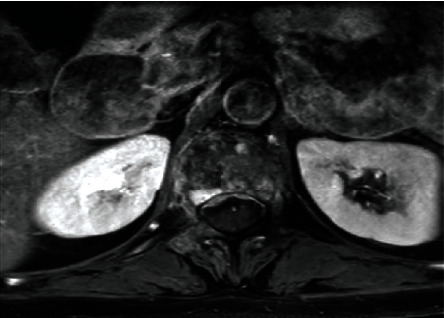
Axial section of an MRI in weighted sequence T1 with gadolinium injection showing irregular contrast uptake of epidural space with heterogeneity of the bone structure vertebral.
